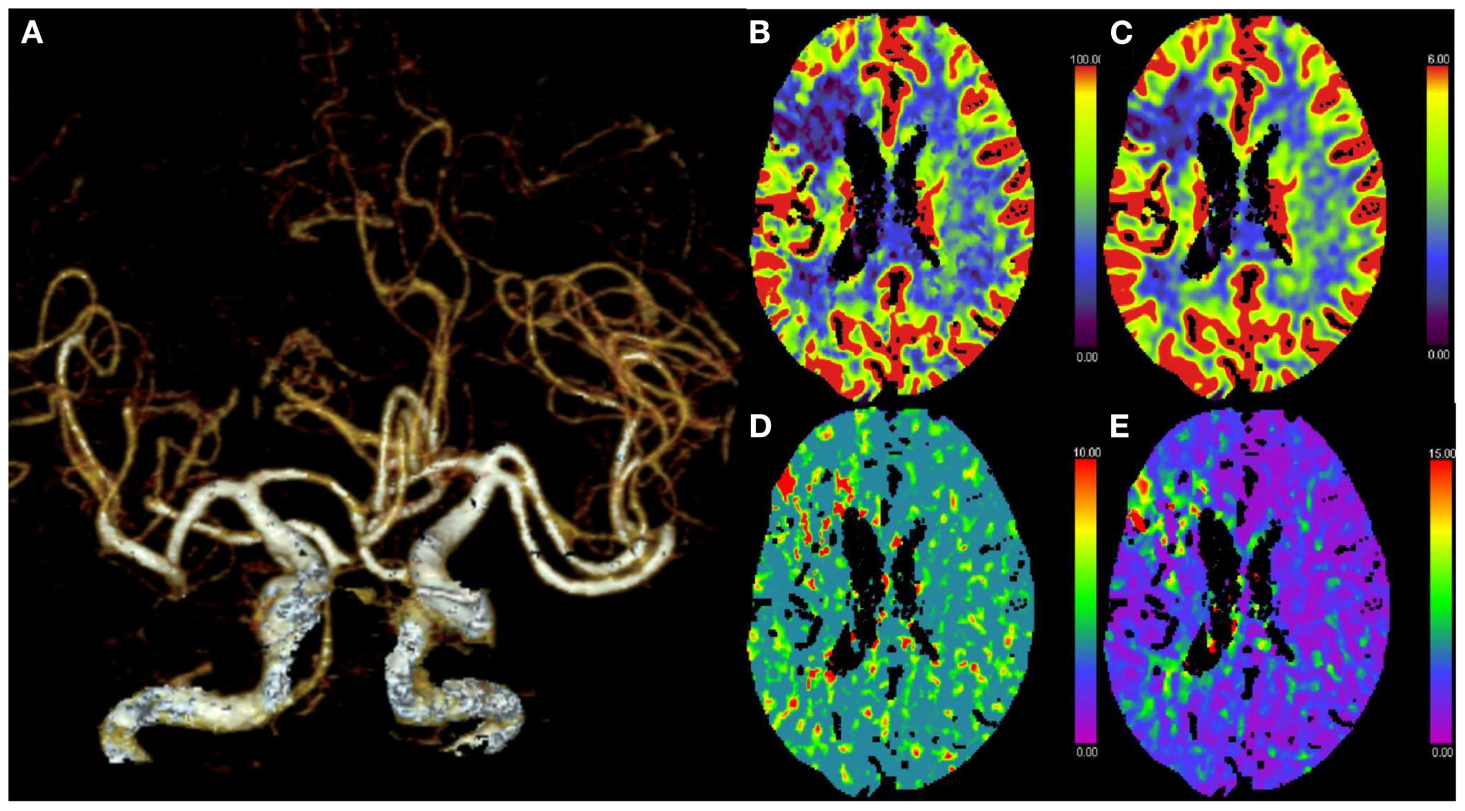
Post-treatment CT angiography (CTA) and perfusion (CTP) at 5 days. (A) CTA demonstrates no occlusion of the ACA and DMCA. (B–E) CTP shows normalization of perfusion in the left cerebral hemisphere.
